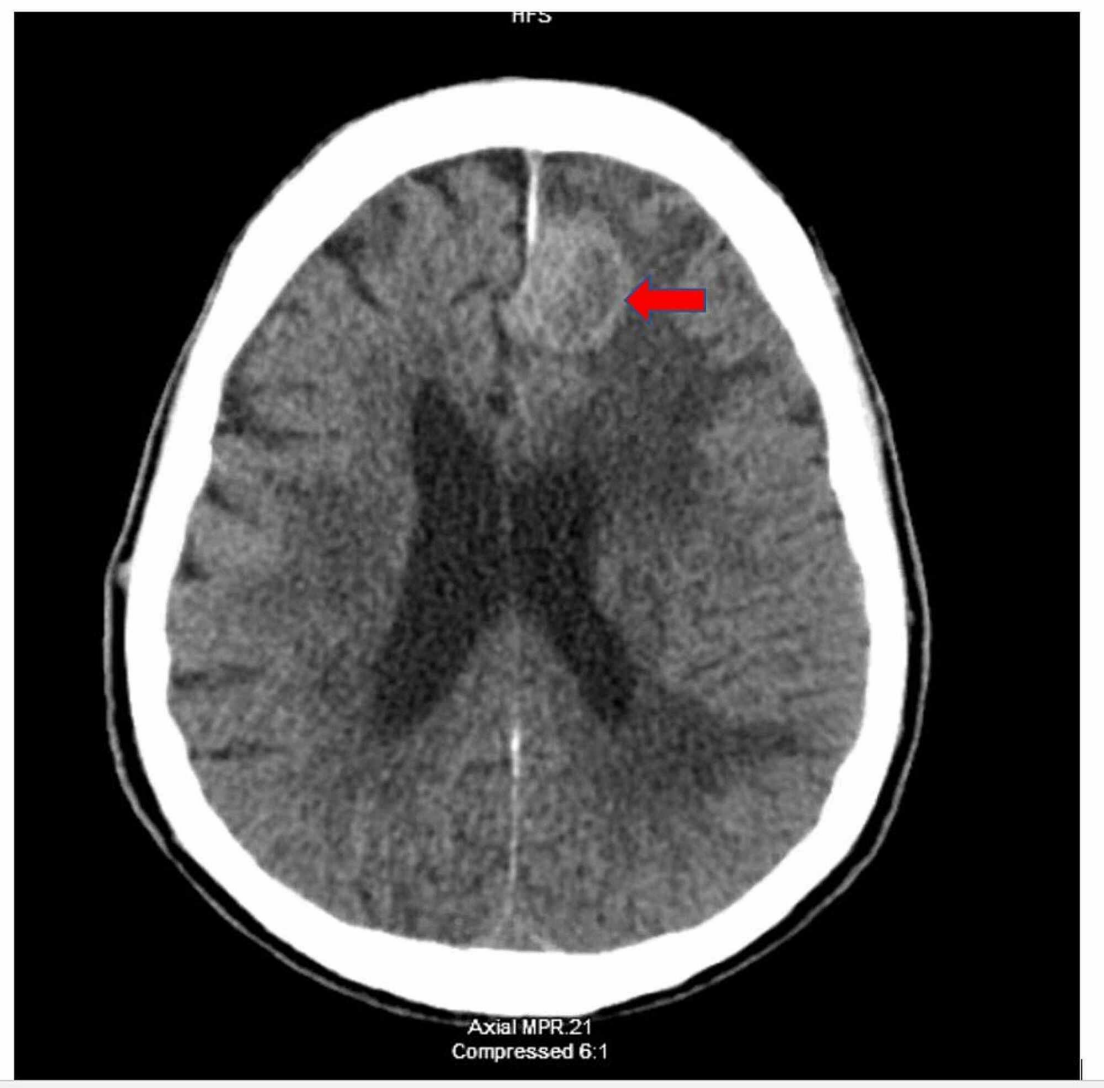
CT brain without contrast, axial section. Red arrow showing frontal lesion with surrounding edema.
